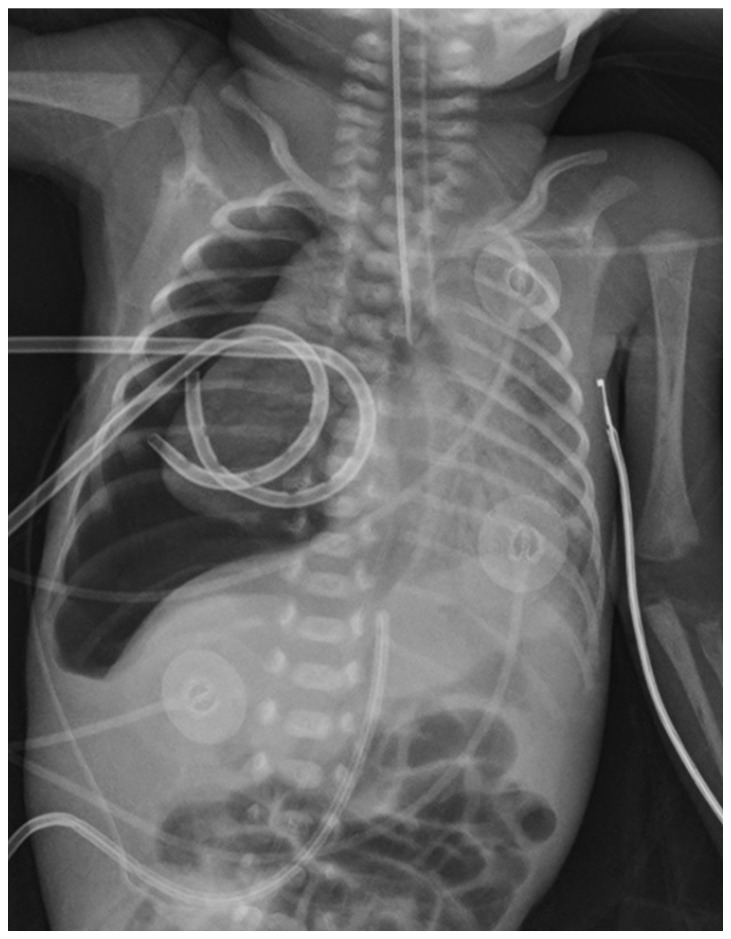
X-ray on the first day of life. Tension right-sided pneumothorax with the leftward shift of the mediastinum after surfactant administration.
